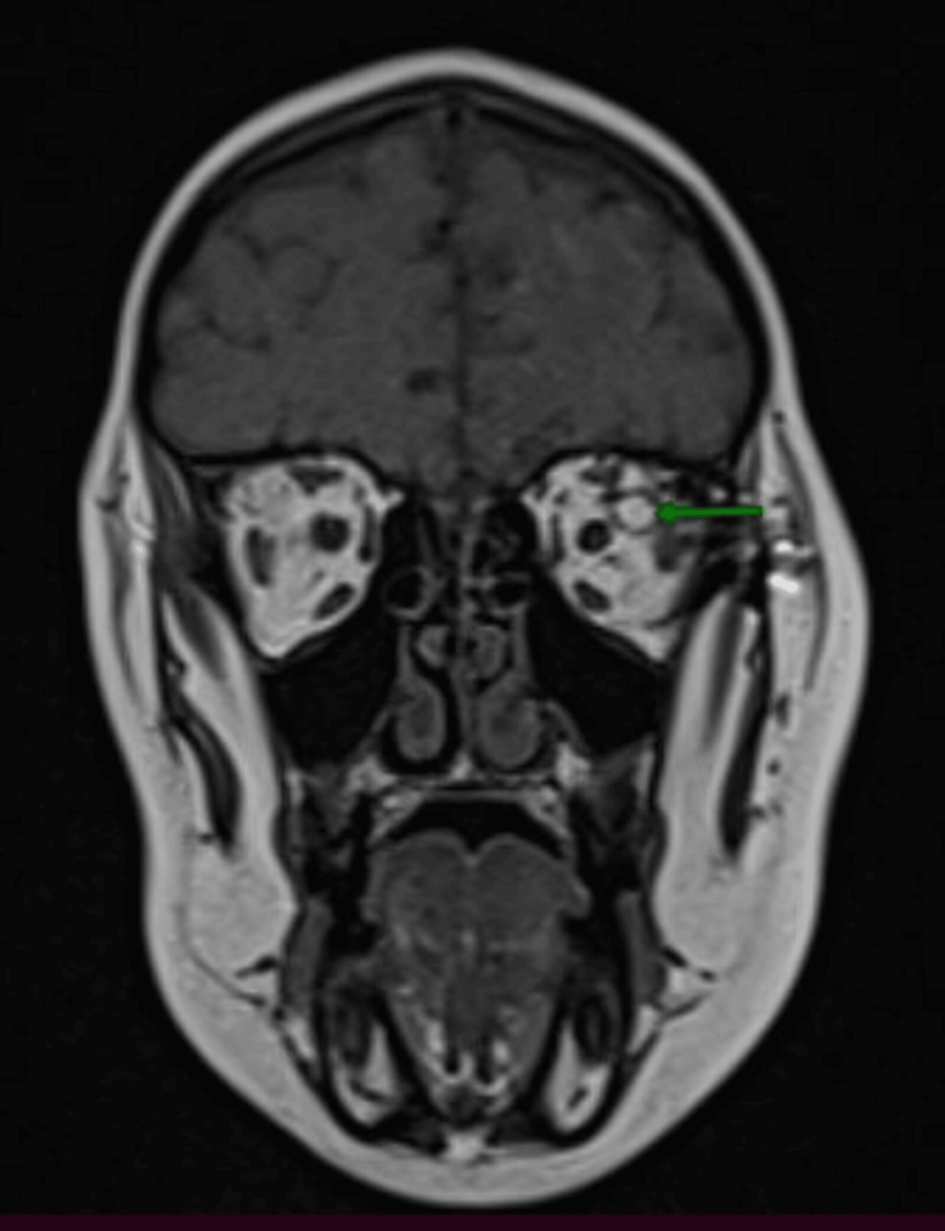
Coronal T1 MRI section revealing a well-circumscribed intraorbital conal mass located at the level of the left superolateral orbital wall, with hypersignal on T1 (green arrow). MRI, magnetic resonance imaging
